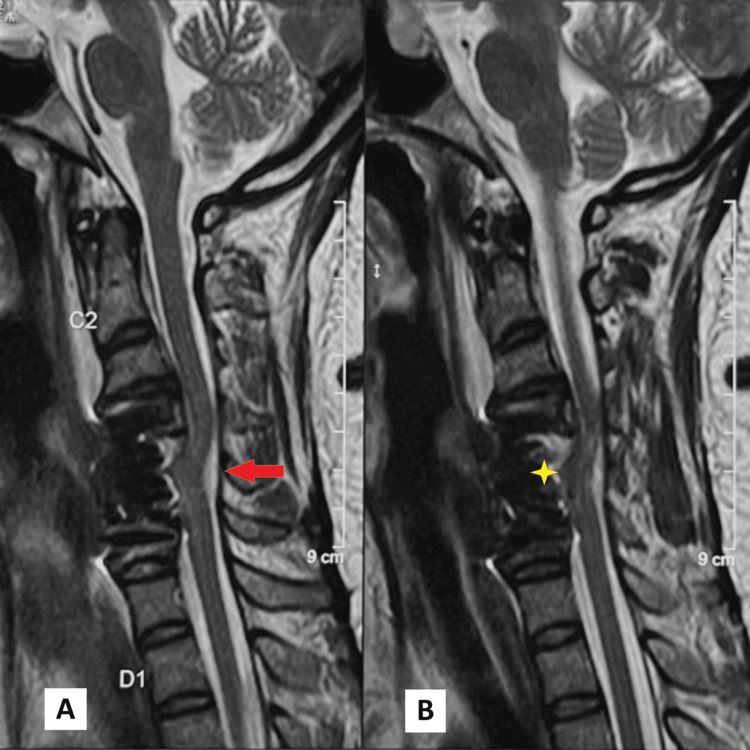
Postoperative day 1 T2w sagittal screening MRI showing ventral cord herniation (VCH) at C5-6 level as suggested by focal anterior angulation of the dura and cord with widened dorsal CSF column (Figure 4A, marked with a red arrow) and tethering of the cord to the anterior aspect of the thecal sac (Figure 4B, marked with a yellow star). MRI: magnetic resonance imaging, T2w: T2-weighted, CSF: cerebrospinal fluid, C5-6: cervical 5-6
